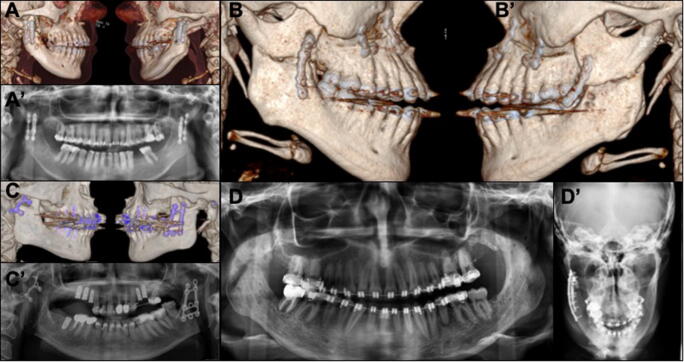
2D images (orthopantomography) and 3D reconstructions (computer tomography) of patients operated on with the high-oblique split osteotomy (HOSO) complicated with failure of the osteosynthesis material. 3D reconstructions show a right and a left view of the patients. (A-A′) Displacement of the proximal segment. (B-B′) Loosening of the osteosynthesis material. (C-C′) Displacement of the proximal segment on the right side. (D) Ramus fracture and pseudarthrosis after the removal of the osteosynthesis material. (D′) Management of the complication by providing new osteosynthesis material
